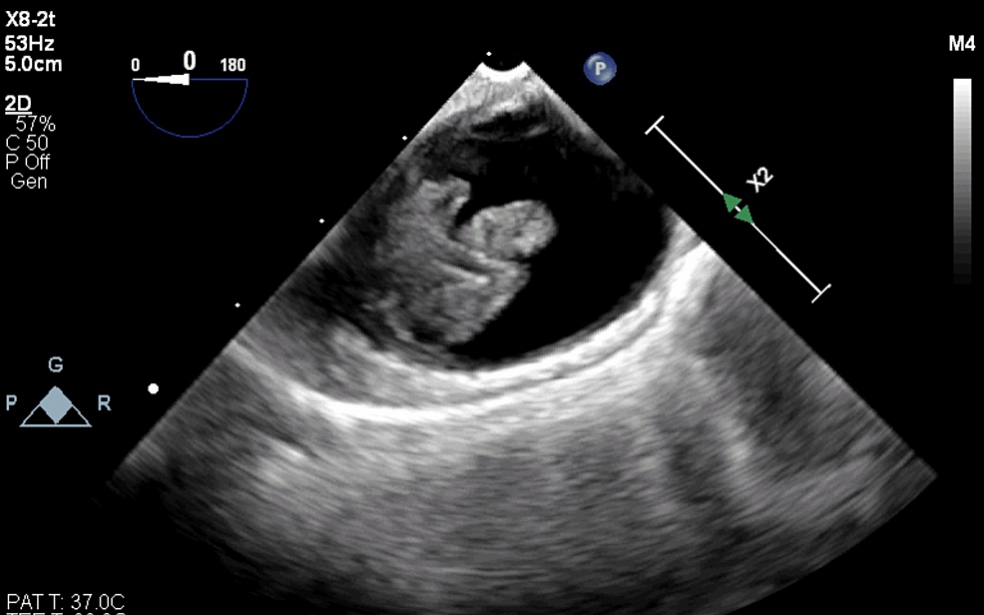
Transesophageal echocardiogram (TEE) confirms the presence of a markedly movable mass located in the distal region of the aortic arch.
